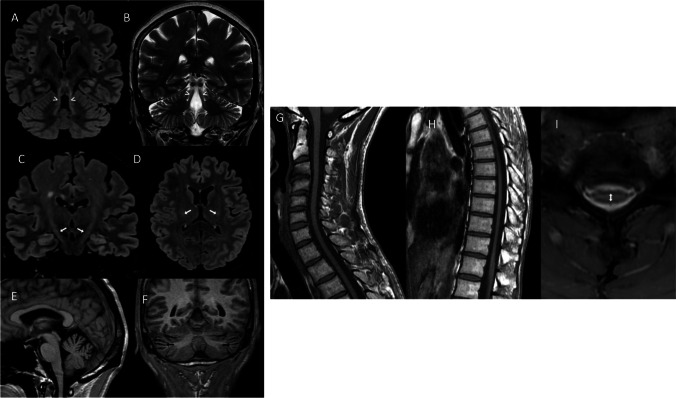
Brain and spinal cord MRI findings in two patients. *Patient 2 A–D*: Hyperintense signal of superior cerebellar peduncles (arrowheads) on coronal 3D T2-FLAIR (A) and T2-weighted (B) sequences; hyperintensity of corticospinal tract (white arrows) on coronal (C) and axial (D) 3D T2-FLAIR sequences. *Patient 9 E–I*: thin corpus callosum (E) on sagittal T1-weighted sequence; cerebellar atrophy (black arrows) on coronal 3D T1-weighted sequence (F). Cervical (G) and thoracic (H) spinal cord atrophy on sagittal T1-weighted and axial MERGE T2-weighted sequences (the latter measured at the level of cervical enlargement—arrow: transverse diameter of 4.3 mm) (I)
